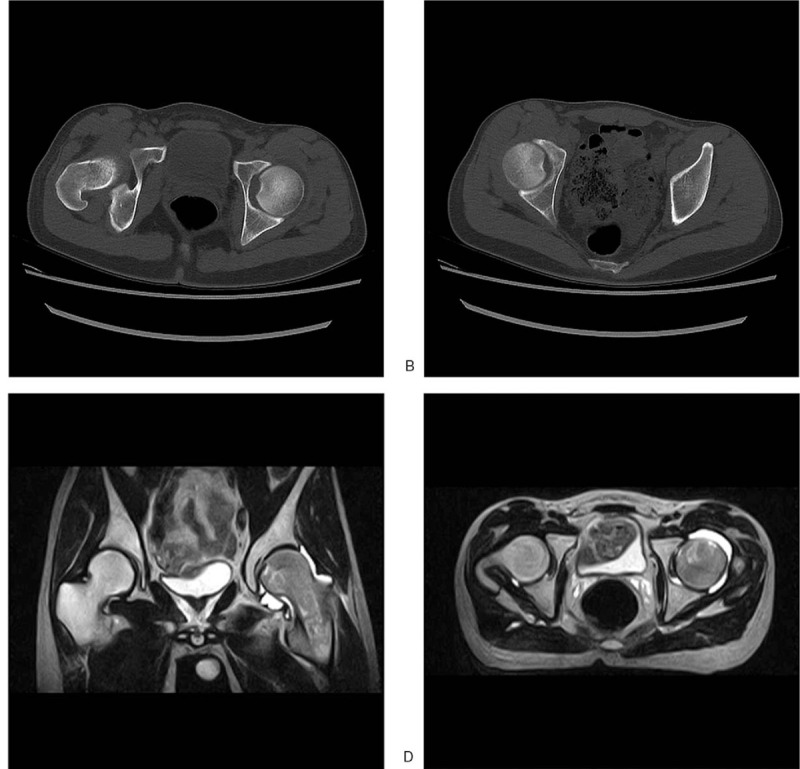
Computed tomography (CT) of the hip joint on July 18, 2016 and magnetic resonance imaging (MRI) of the hip joint on July 19, 2016. *A and B*, CT shows the bilateral hip joint space is narrowed, the articular surface is still intact, the bone trabecular patchy density under the articular surface of the right femoral head is increased uniformly, effusion can be seen in the left hip joint capsule and the left femoral head has no obvious abnormalities. Right femoral head ischemic necrosis and left hip joint effusion were considered. *C and D*, MRI shows the position of the hip joint is normal, the joint space is narrowed, the signal of articular cartilage is not uniform, and the articular surface is intact. The signal of the left femoral head, femoral neck and upper femur is not uniform. The signal of the left pubic muscle and external obturator muscle, local iliopsoas muscle and lateral femoral muscle is slightly increased. Under the cartilage of the right femoral head, patchy long T1 and long T2 and high lipid pressure signal were observed. No abnormal signals were found in the bilateral acetabulum, right femoral neck and upper femur. The T1 signal of bone seen in the scanning range decreased uniformly and the T2 lipid pressure showed uniform high signal. Fluid signals were seen in the pelvis.
